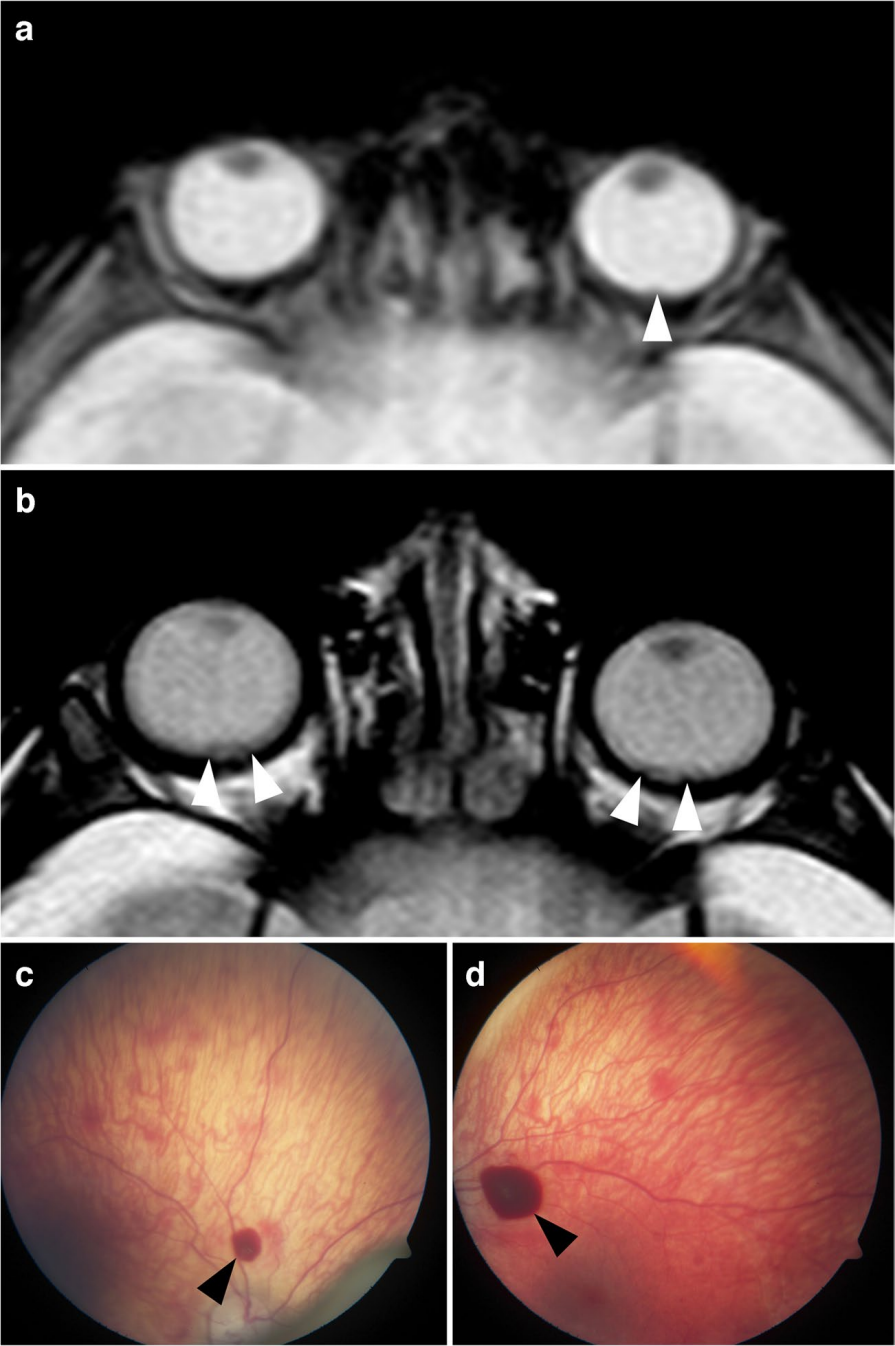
A 5-month-old girl with abusive head trauma following shaking and bilateral retinal hemorrhages detected in magnetic resonance imaging as well as in fundoscopy, both conducted on the same day. a Axial T2*-weighted magnetic resonance image through the orbits demonstrates a small retinal hemorrhage at the posterior pole of the left eye (*arrowhead*). b Axial T2-weighted image through the orbits shows irregularities at both posterior poles consistent with retinal hemorrhages (*arrowheads*). c, d Fundoscopy images of both eyes show delicate, diffuse subretinal hemorrhages across the entire fundi. Additionally, disc-sized epiretinal hemorrhages are visible along the major vascular arcades (*arrowheads*)
